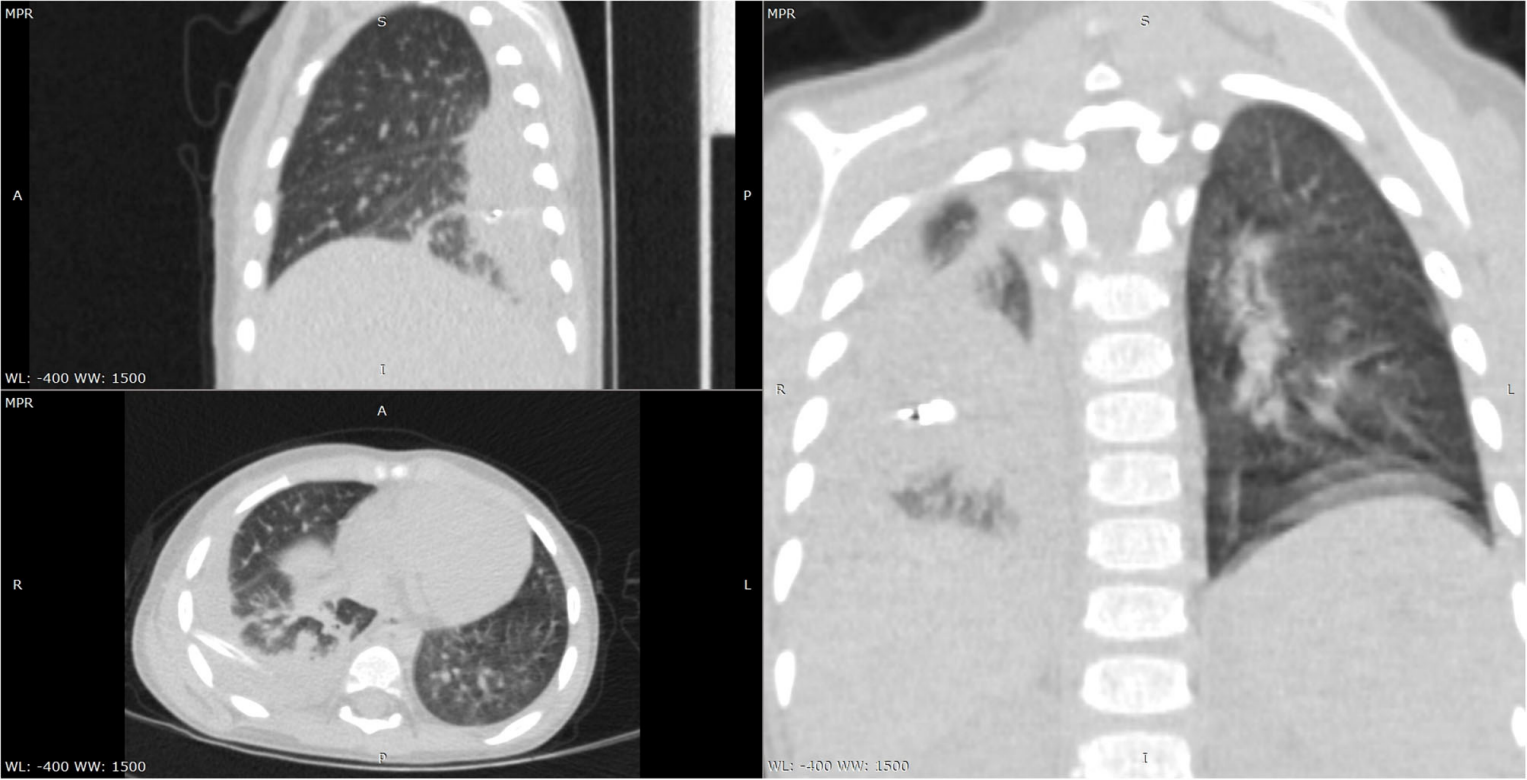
Chest CT scan—multiplanar reconstructions lung window-encapsulated pleural effusion with drain tube.
